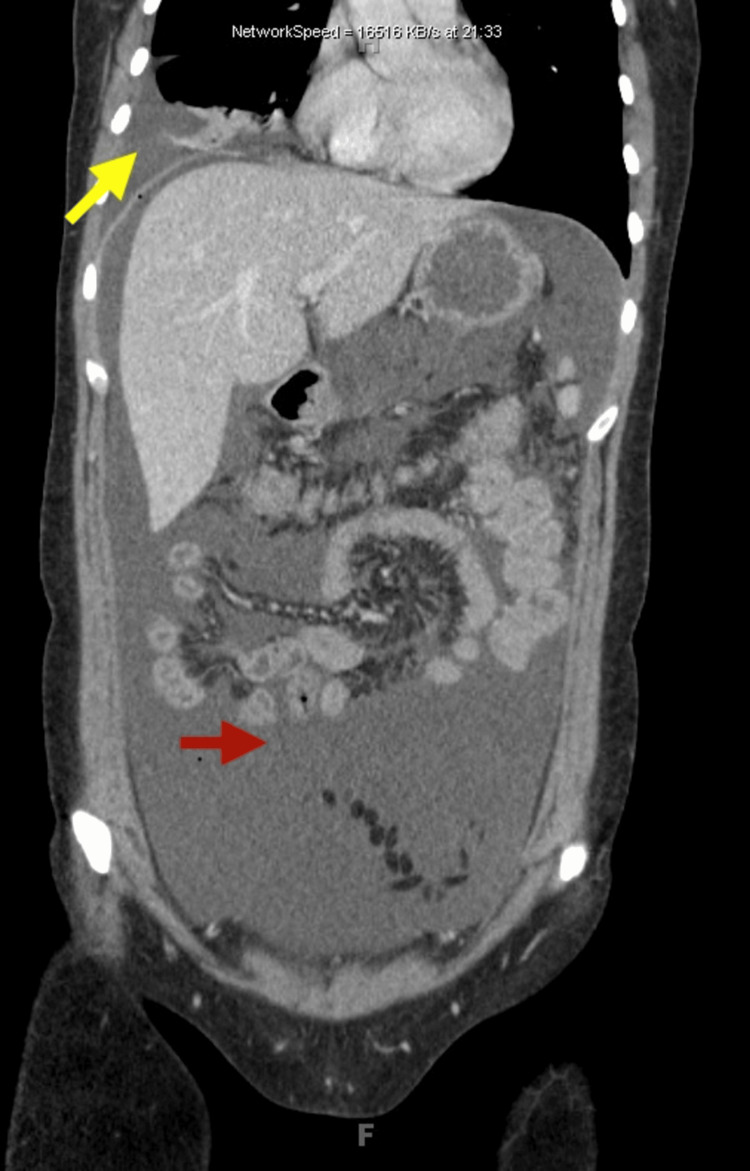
CT scan of the chest, abdomen, and pelvis This CT scan shows a pleural effusion (indicated by the yellow arrow) and ascites (indicated by the red arrow).
